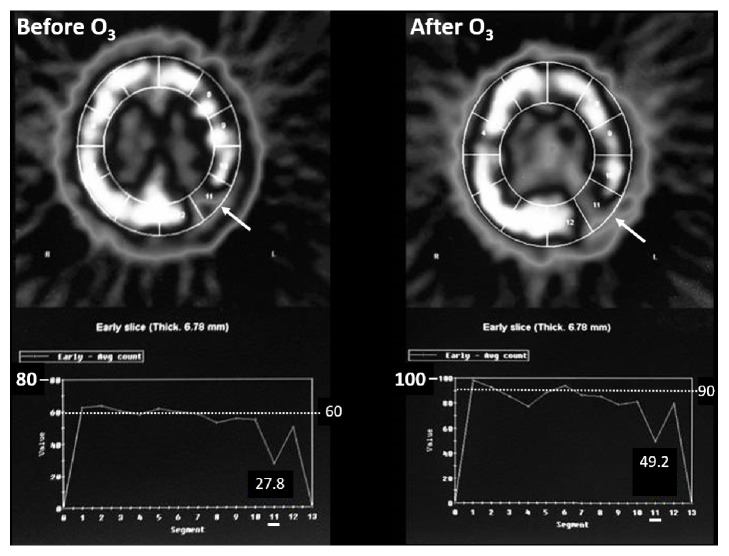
Ozone therapy and cerebral blood flow assessed by SPECT-ECD. Cerebral blood flow assessed by single photon emission computed tomography (SPECT) with ECD (^99m^Tc-ethyl cysteinate dimer); the tracer correlates with cerebral blood flow. The figure depicts a 68-year-old patient with a left parietooccipital glioblastoma (astrocytoma Grade IV) following subtotal resection. SPECT with ECD was carried out before (*Left*) and after 3 O_3_T sessions on alternate days (*Right*). After 3 sessions of O_3_T, (1) overall SPECT-index in brain increased from 60% to 90% and (2) in the tumor area (section #11) SPECT-index increased from 28% to 49%, increase >50%. Note that there are different scales before and after O_3_T.
